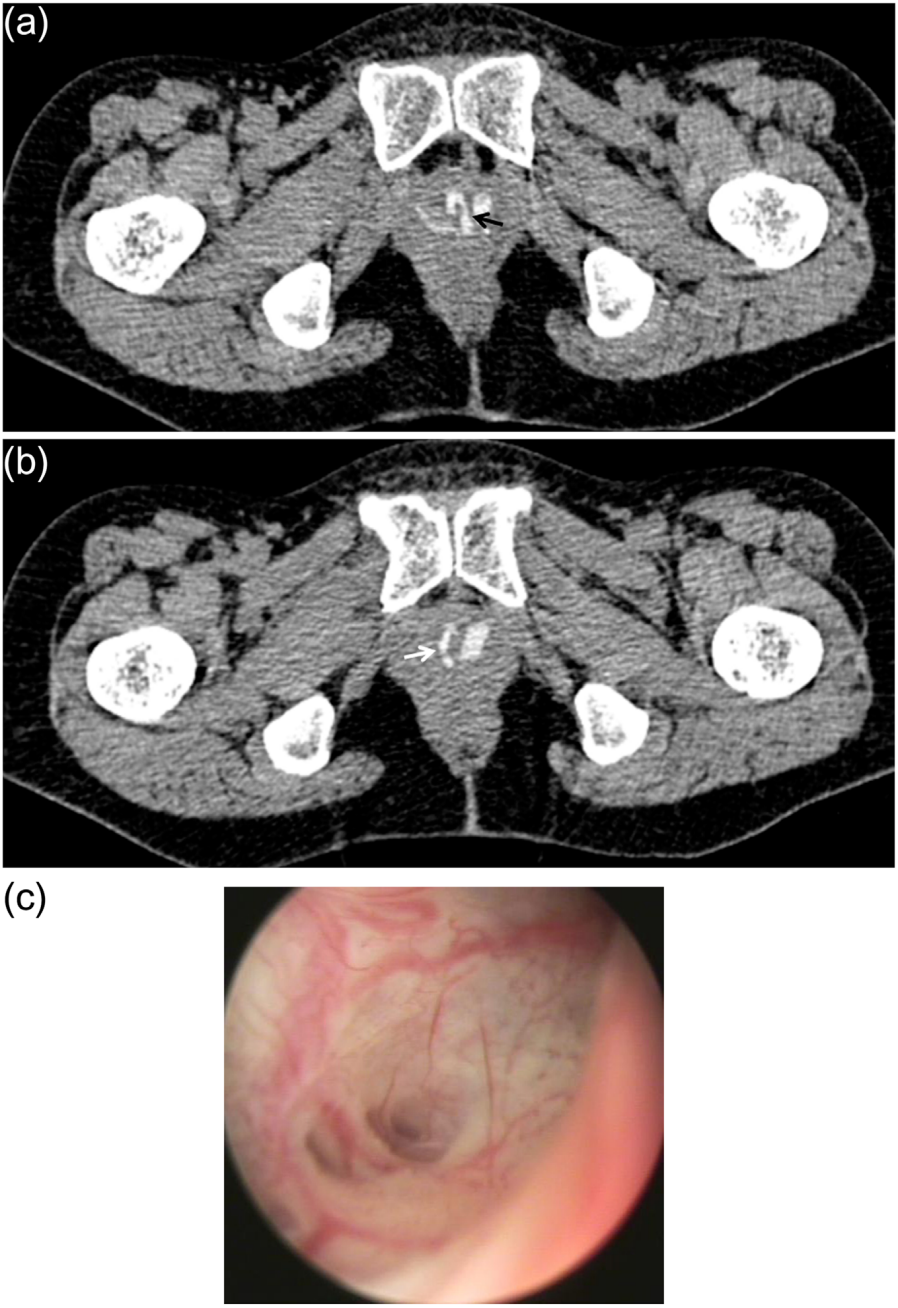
Comparison of CT-VCUG and cystourethroscopy showing two ostia. Forty-nine year old female presented with recurrent cystitis. The axial image of CT-VCUG showed the diverticular ostia (a) at 5 (black arrow) and (b) 7 o’clock (white arrow) direction. (c) Cystourethroscopy of the same patient revealed an opening under direct vision at the location as predicted by CT-VCUG.
